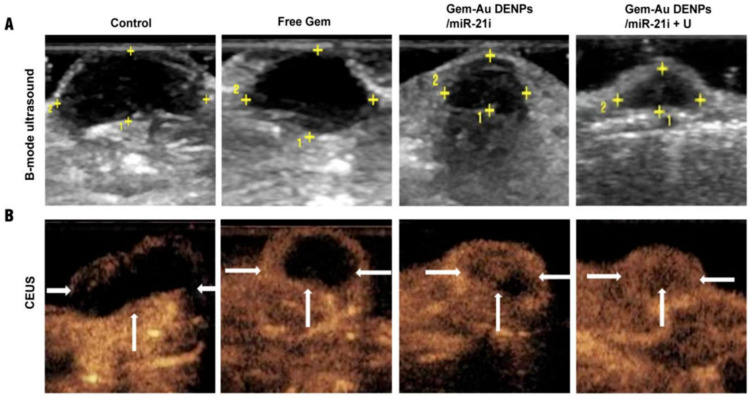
(A) shows a B-mode ultrasound image, with the yellow plus signs indicating the tumor region. (B) shows a contrast-enhanced ultrasound (CEUS) image is presented. The CEUS imaging reveals abundant intra-tumoral blood flow and a smaller tumor volume in the Gem-Au DENPs/miR-21i + U group. Adapted with permission from [136].
